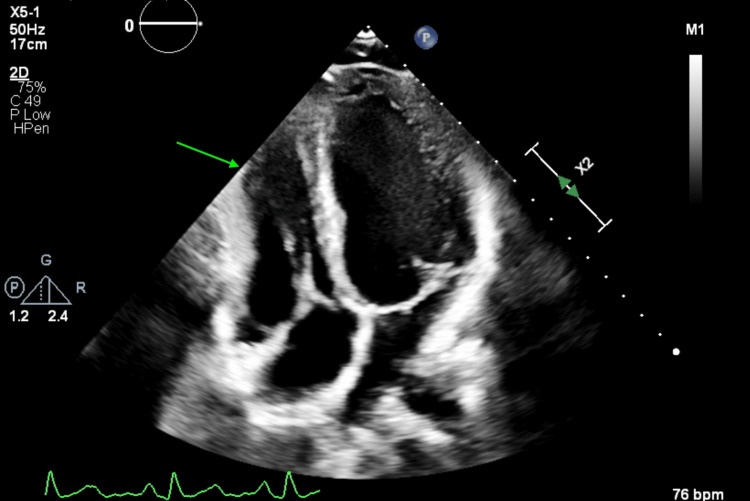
Apical four-chamber transthoracic echocardiographic view demonstrating distortion and focal compression of the right ventricular free wall. Quantitative measurements include right ventricular S’ velocity of 12 cm/s and right ventricular diastolic basal dimension of 4.2 cm. Left ventricular biplane with an ejection fraction of 47%. Arrow highlights the region of altered right ventricular geometry consistent with external compression from severe pectus excavatum.
